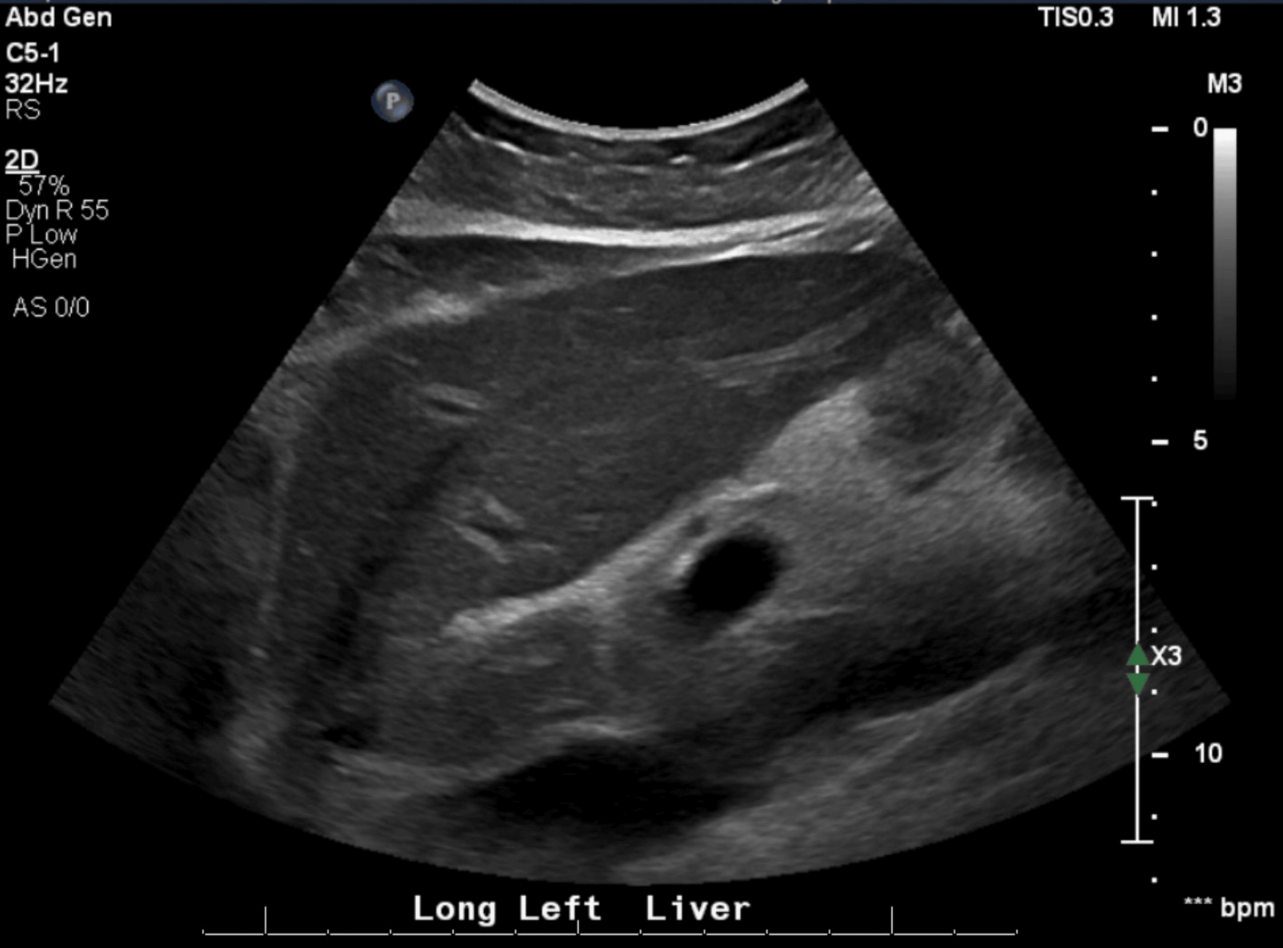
Right upper quadrant ultrasound demonstrating no biliary obstruction Right upper quadrant ultrasound image demonstrating no gallstones, no sonographic evidence of acute cholecystitis, and no intrahepatic or extrahepatic biliary ductal dilatation, supporting a non-obstructive etiology for the patient’s transaminitis.
